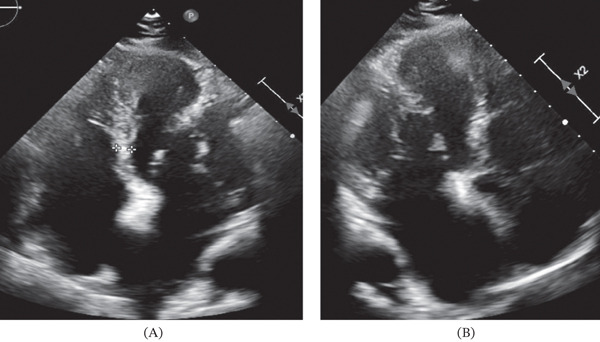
Transthoracic echocardiography—(A) Apical four‐chamber view showing a prominent anterolateral papillary muscle and (B) apical two‐chamber view showing a prominent anterolateral papillary muscle.
